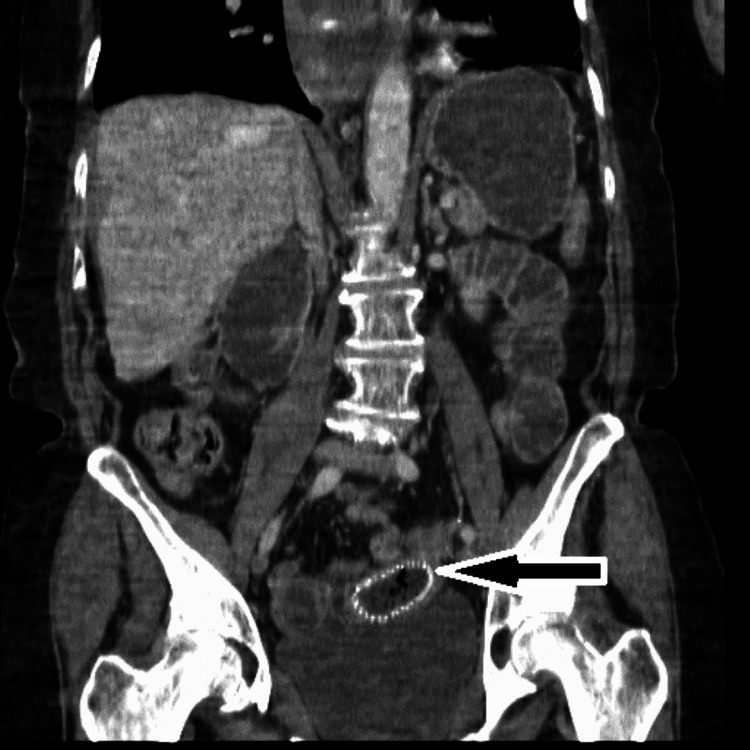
Coronal CT demonstrating the stent (black arrow) located within the small bowel. CT: computed tomography
